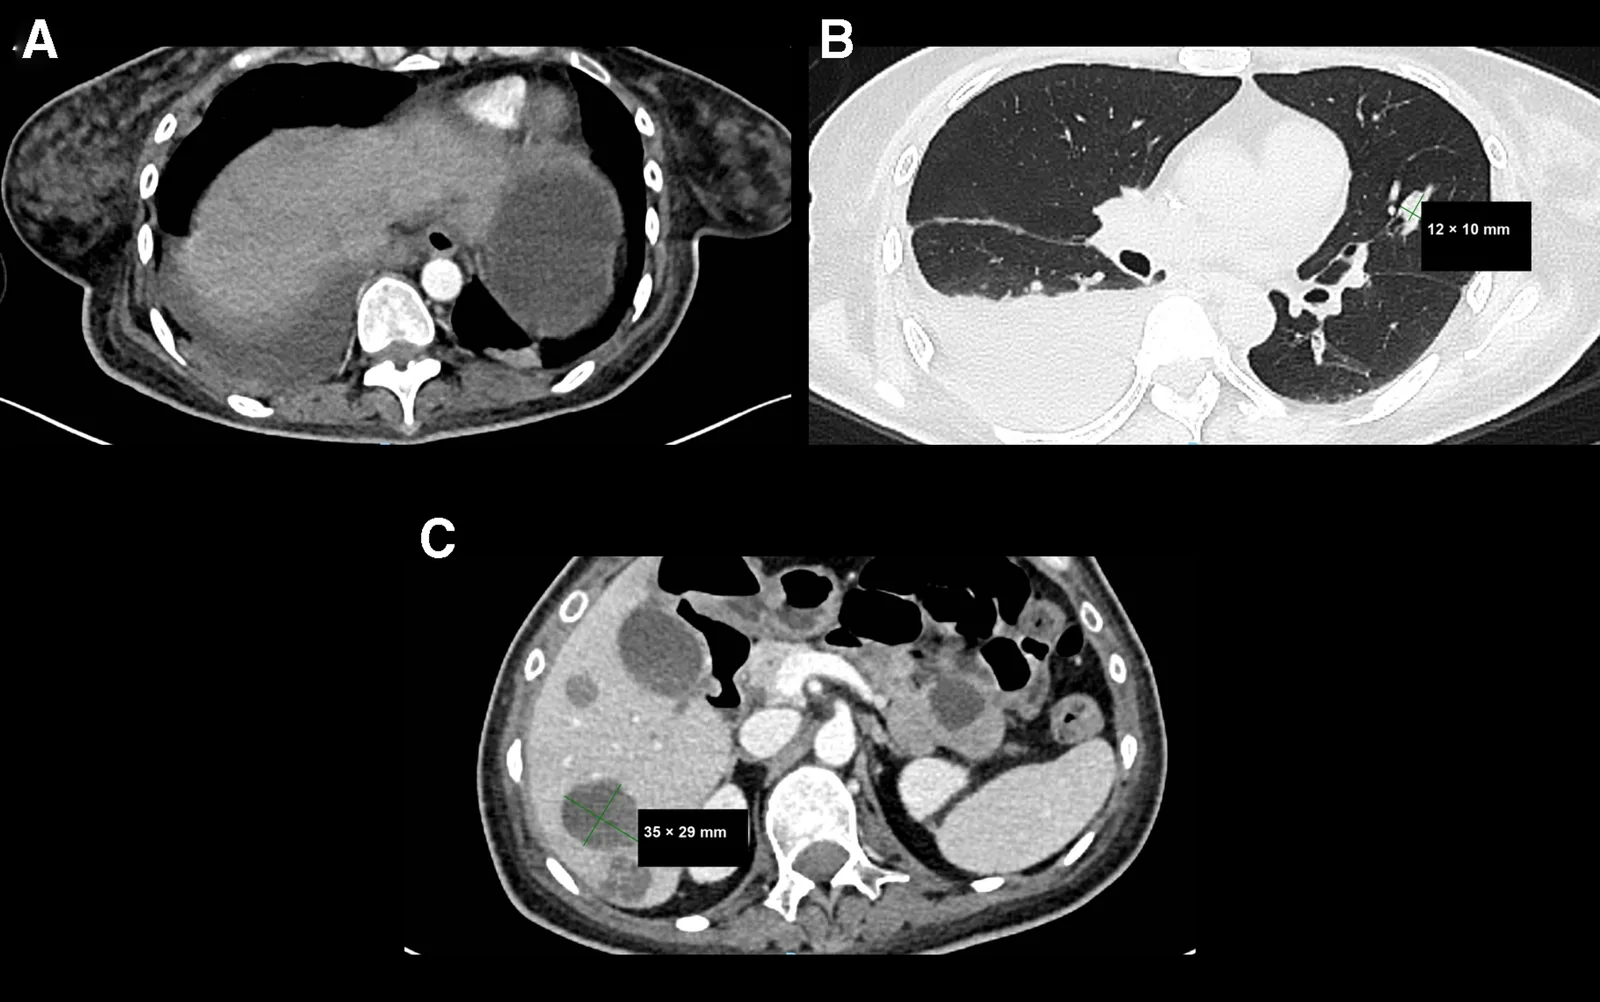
Thoracic and abdominal metastatic disease on CT obtained on March 11, 2024. (A) Large right pleural effusion with adjacent compressive atelectatic change. (B) A left pulmonary nodule measuring approximately 12 × 10 mm. (C) Multiple hepatic lesions, including a representative lesion measuring approximately 35 × 29 mm. These measurements represent disease burden on this examination and were not used as paired treatment-response measurements. CT = computed tomography.
